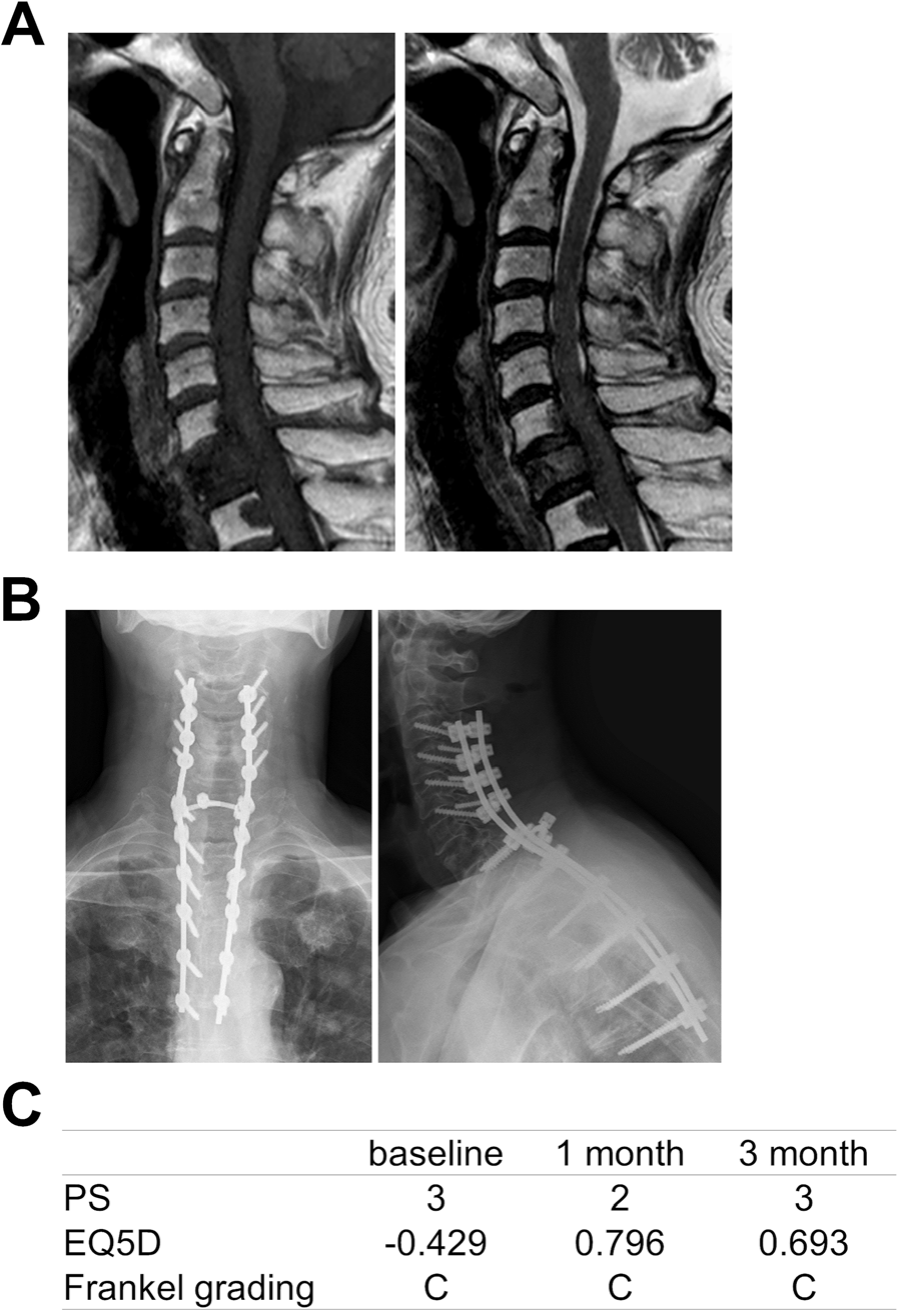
Images and clinical course of the 69-year-old man with metastatic hepatocellular carcinoma to the C7 vertebra. A Preoperative MRI: sagittal T1-weighed enhanced image at the left side and sagittal T2-weighed enhanced image at the right side. B Postoperative radiographs: posteroanterior image at the left side and lateral image at the right side. C PS, EQ5D, and Frankel grading preoperatively and at 1 and 3 months postoperatively. MRI, magnetic resonance imaging; PS, performance status; EQ5D, EuroQol 5-Dimension
